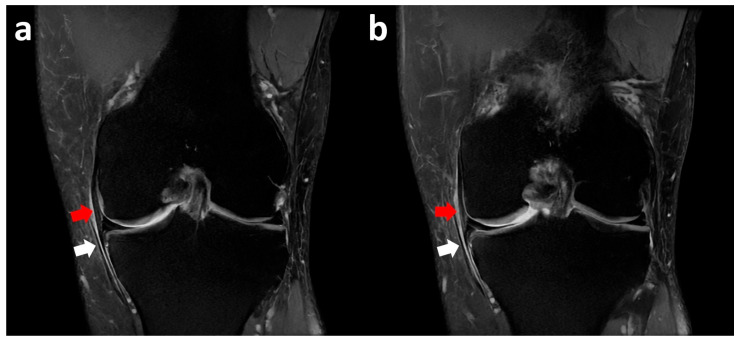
Left knee coronal fat-suppressed PD MR images show a medial meniscus body tear (a) with an open oblique course on the inferior side associated with a meniscal flap (white arrows) displaced inferiorly and trapped between the medial tibial bony surface and medial collateral and capsular–ligamentous complex (red arrows) (b).
